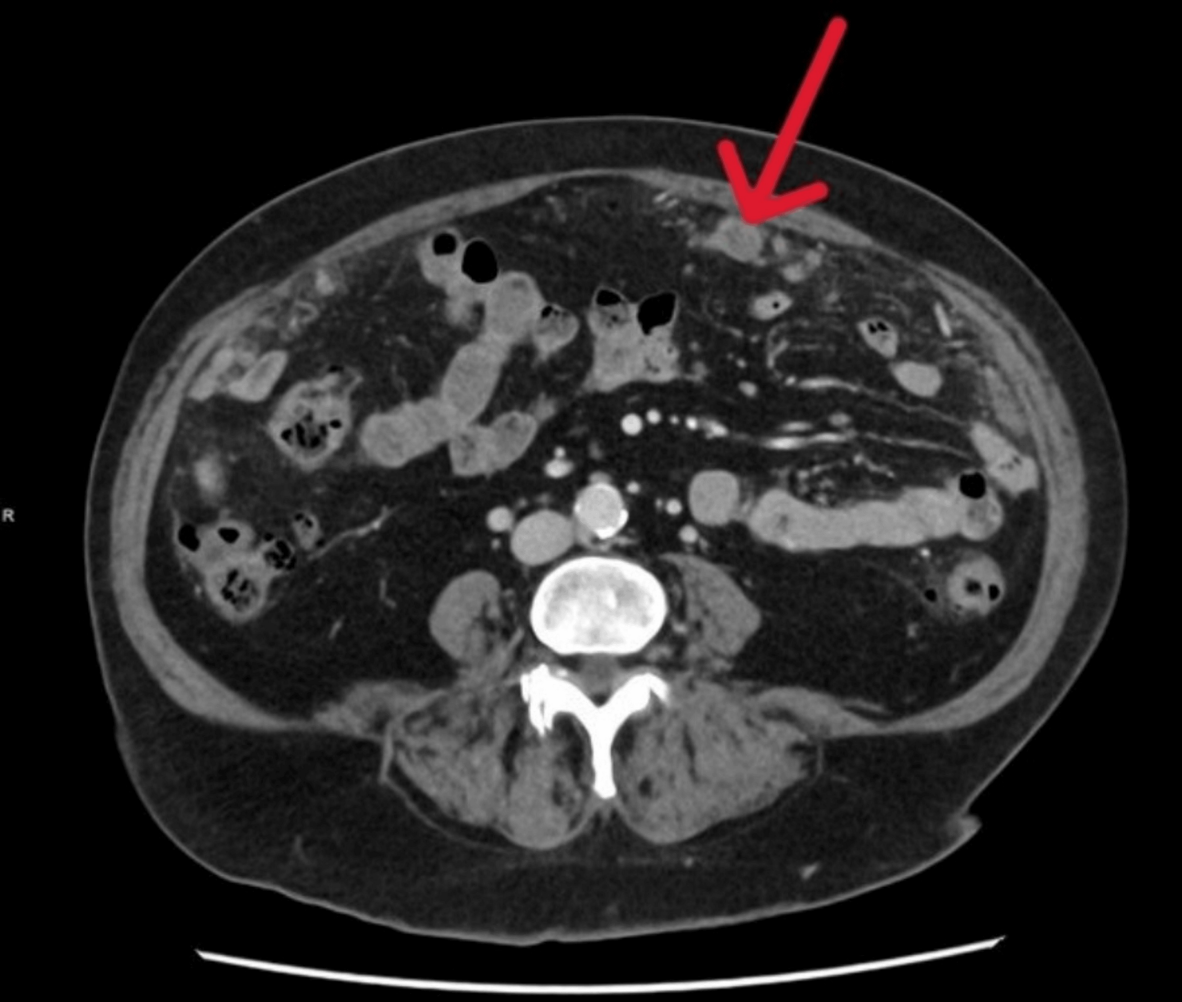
Axial CT image from a different patient demonstrating peritoneal carcinomatosis, included for comparison. The irregular soft-tissue peritoneal implants and omental involvement resemble the cystic lesions in this case (arrow), highlighting the potential for peritoneal inclusion cysts to mimic malignant disease. This image is from a different patient and has been fully de-identified in accordance with institutional and ICMJE privacy standards. ICMJE: International Committee of Medical Journal Editors
